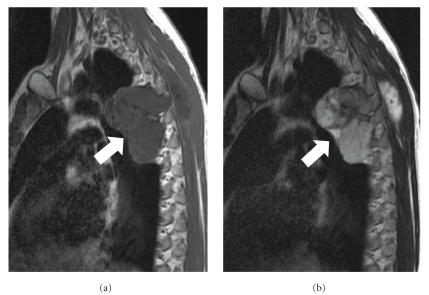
MR imaging of the same patient. The sagittal T1-w (a) MR image shows a hypointense lobulated lesion (arrow). (b) The sagittal T2-w MR image shows the lesion with heterogeneous but predominantly high signal intensity (arrow). Note the superficial palpable component of the tumor (asterisks).
